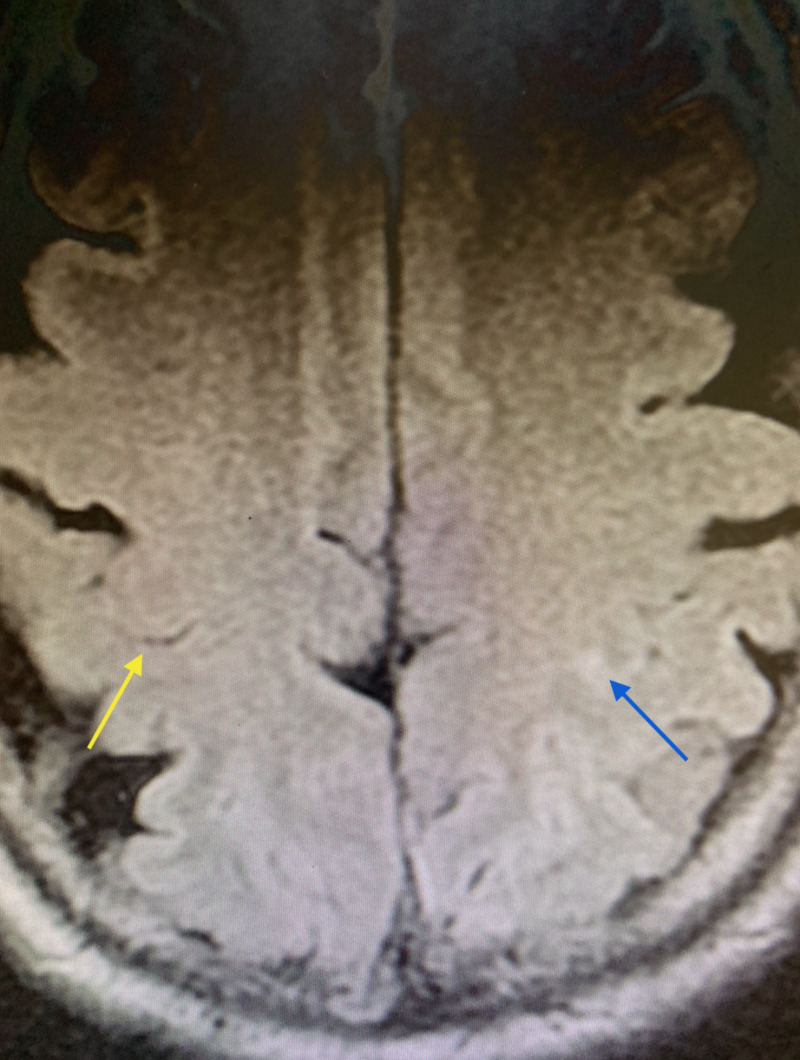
FLAIR sequence: 0.3 tesla MRI Right omega sulcus (yellow arrow), hyperintensity left-hand knob gyrus obscuring omega sulcus (blue arrow) MRI: magnetic resonance imaging; FLAIR: fluid-attenuated inversion recovery
